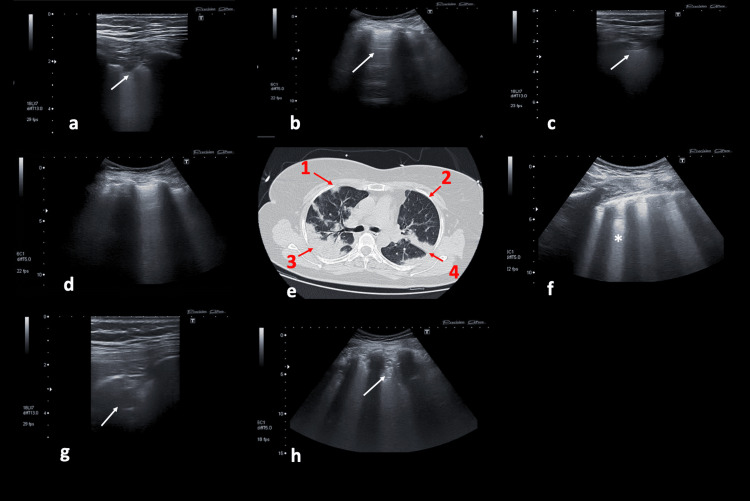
The images of LUS and CT of the patients with COVID-19 pneumonia The a-d, g-h, and c-f are the examinations of the same area via a different probe. (a, b, c, d, f, g, h) LUS. (e) CT. a. Subpleural consolidation (white arrow) was obtained with the linear probe, LUS score 2, corresponding to area 1 (red arrow) on CT. b. In the image, 'A lines' (white arrow) are present. This image was evaluated as LUS score 0. This image corresponds to area 2 on CT. c. The normal appearance of the pleural line has disappeared and there is major consolidation. This image was evaluated as LUS score 3. It corresponds to area number 4 in CT. d. B lines are more than 3. LUS score 1, corresponding to area 1 on CT. e. CT. g. The consolidation image was examined with a linear probe, corresponding to area 3 on CT. h. The consolidation pattern obtained when examined with a convex probe, LUS score 3, corresponding to area 3 on CT. f. White lung (*) image obtained when examined with a convex probe, LUS score 3, corresponding to area 4 on CT. CT, computed tomography; LUS, lung ultrasonography
